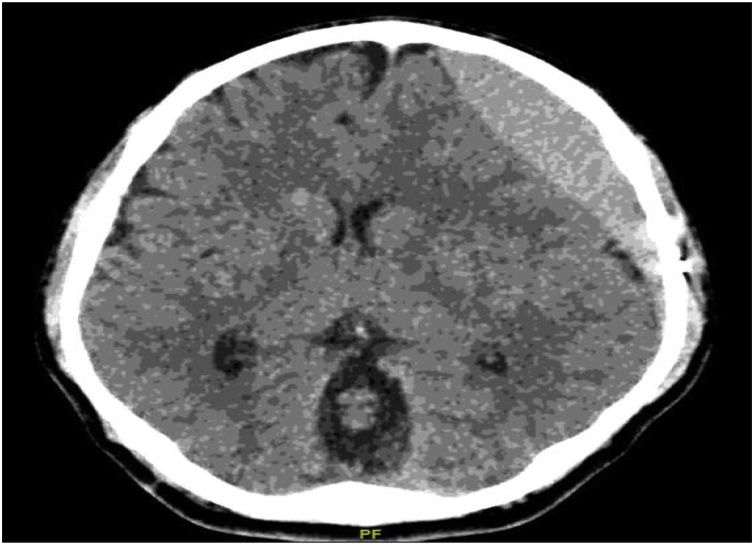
CT brain following the second presentation showing recollection of hematoma in the left subdural space.
